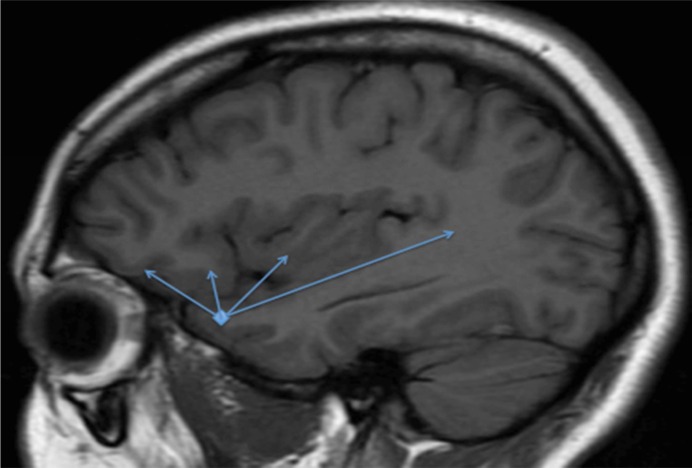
Sagittal magnetic resonance imaging (MRI) of the head
